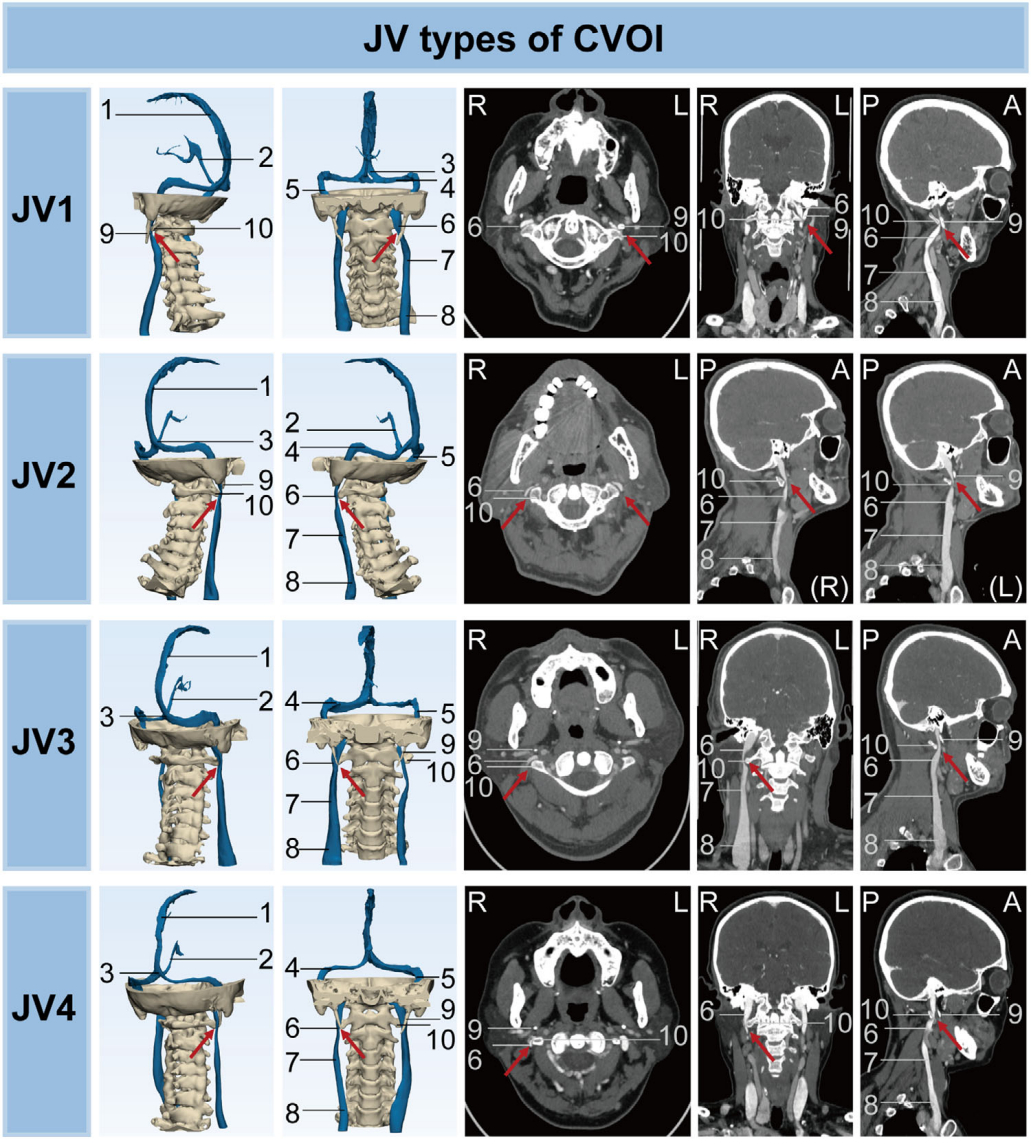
Imaging characteristics of JV types of CVOI on CT. Numbered labels: 1 = superior sagittal sinus, 2 = inferior sagittal sinus, 3 = confluence of sinuses, 4 = transverse sinus, 5 = sigmoid sinus, 6 = internal jugular vein (J3 segment), 7 = internal jugular vein (J2 segment), 8 = internal jugular vein (J1 segment), 9 = styloid process, 10 = transverse process of the atlas (C1). CVOI, cerebral venous outflow insufficiency; JV, extracranial type of CVOI; R, right; L, left; P, posterior; A, anterior.
